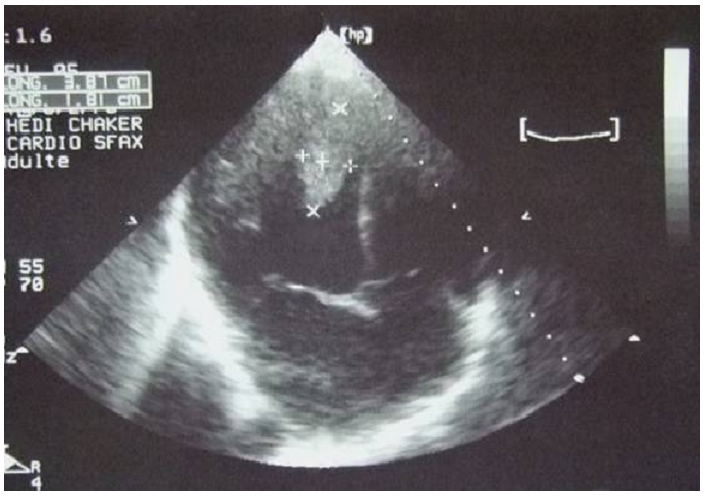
Four-chamber view echocardiography of a young patient with antiphospholipid syndrome and myocardial infraction showing an apical large thrombus measuring 38x18 mm
